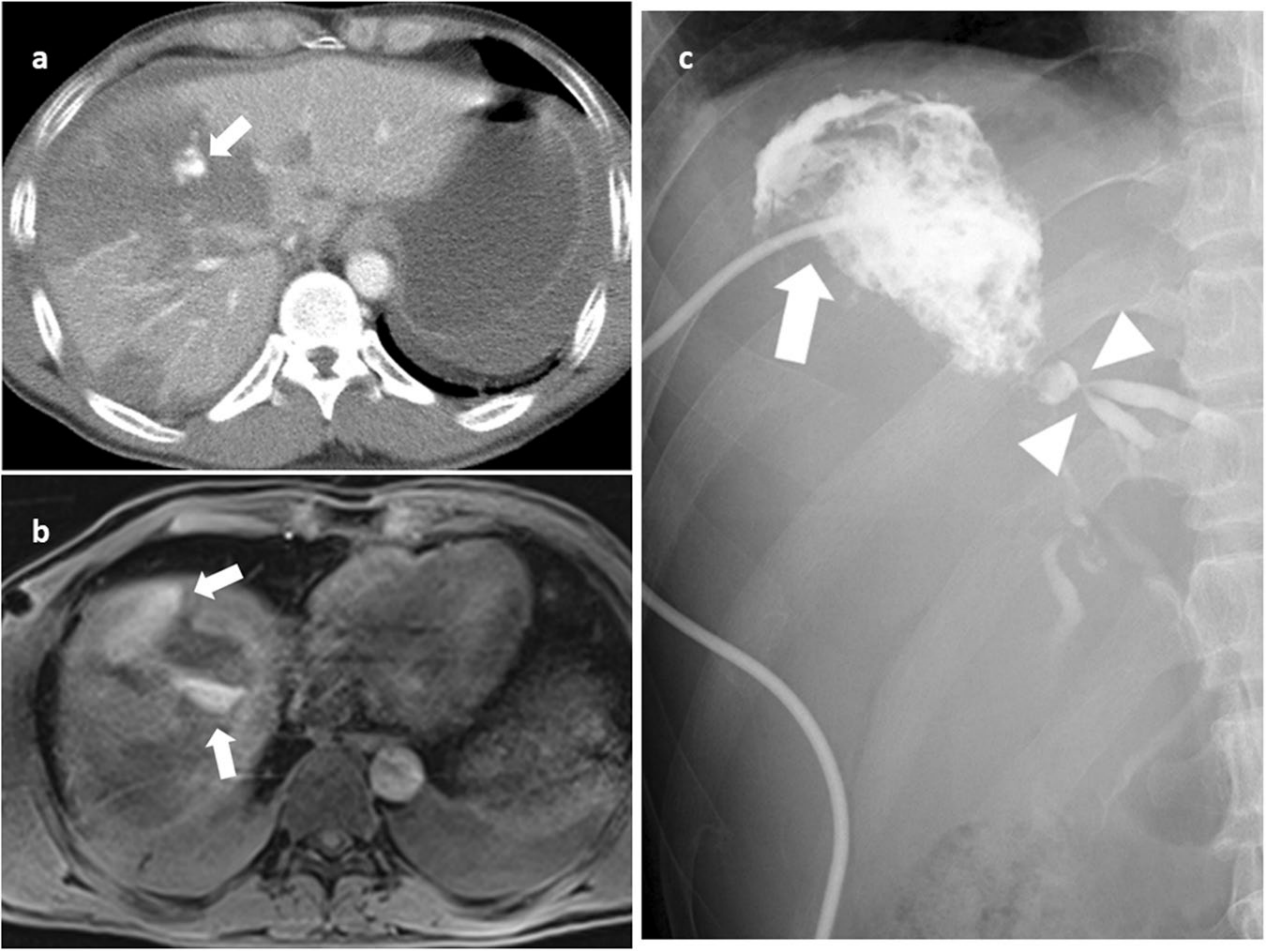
CT, MRI and cavitrography of a 36-year-old man with grade IV liver injury. (a) Contrast-enhanced CT in axial plane shows active extravasation of contrast medium (arrow) at the liver parenchyma, treated initially with angioembolization (not shown). (b) Contrast enhanced MR cholangiography acquired at 90 minutes in axial plane shows a type II bile leak (arrows) expanding through the disrupted anterior liver capsule to perihepatic space. (c) Percutaneous cavitography through a pigtail catheter (arrow) shows a direct communication of the injected iodinated contrast medium (biloma) with the injured intrahepatic bile ducts (arrowheads). He was treated with both percutaneous catheter drainage and endoscopic retrograde biliary drainage and later developed intrahepatic bile duct stricture.
